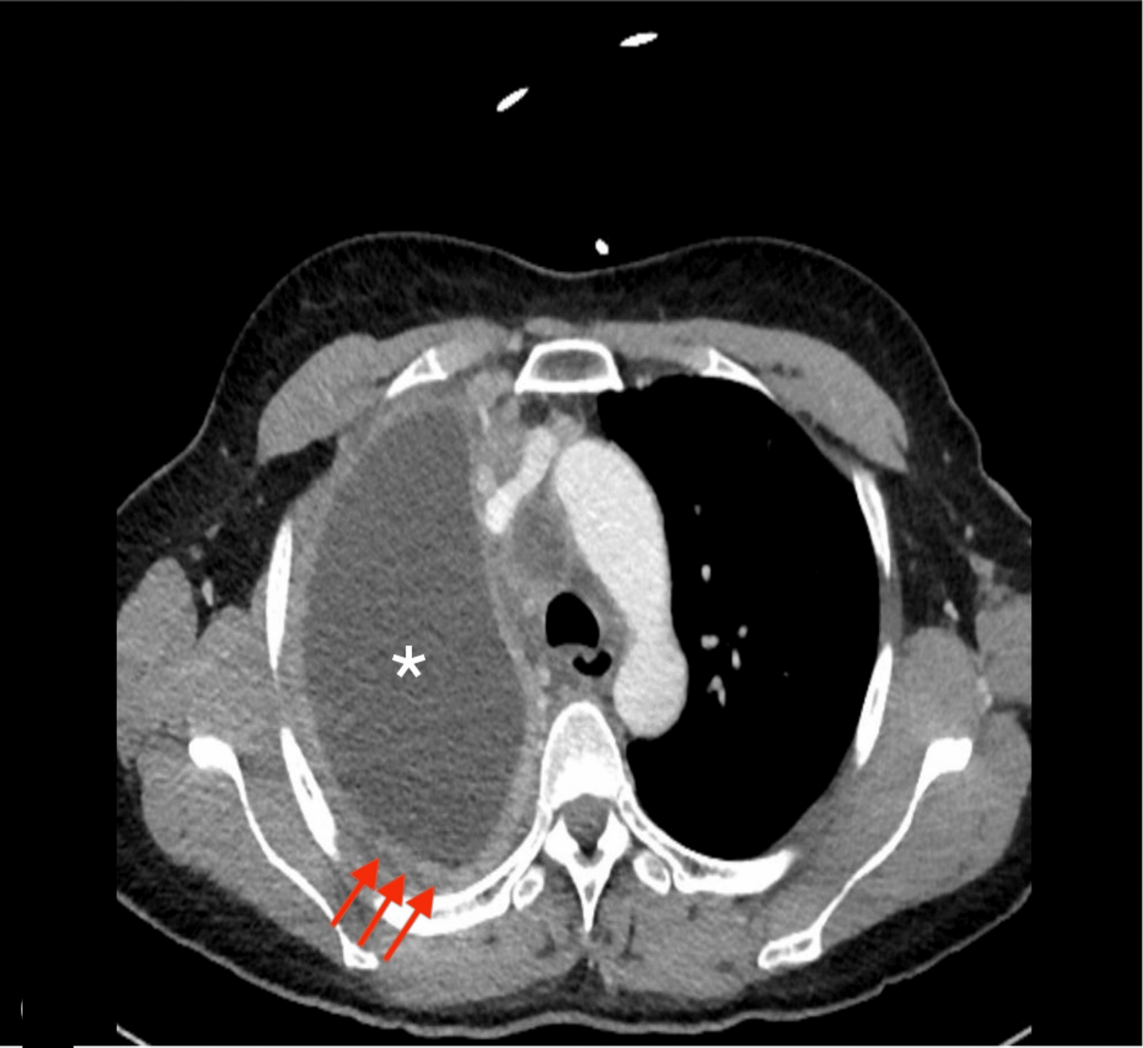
CT Chest with contrast axial view: irregular pleural thickening (red arrows) and pleural effusion (white asterisk)
